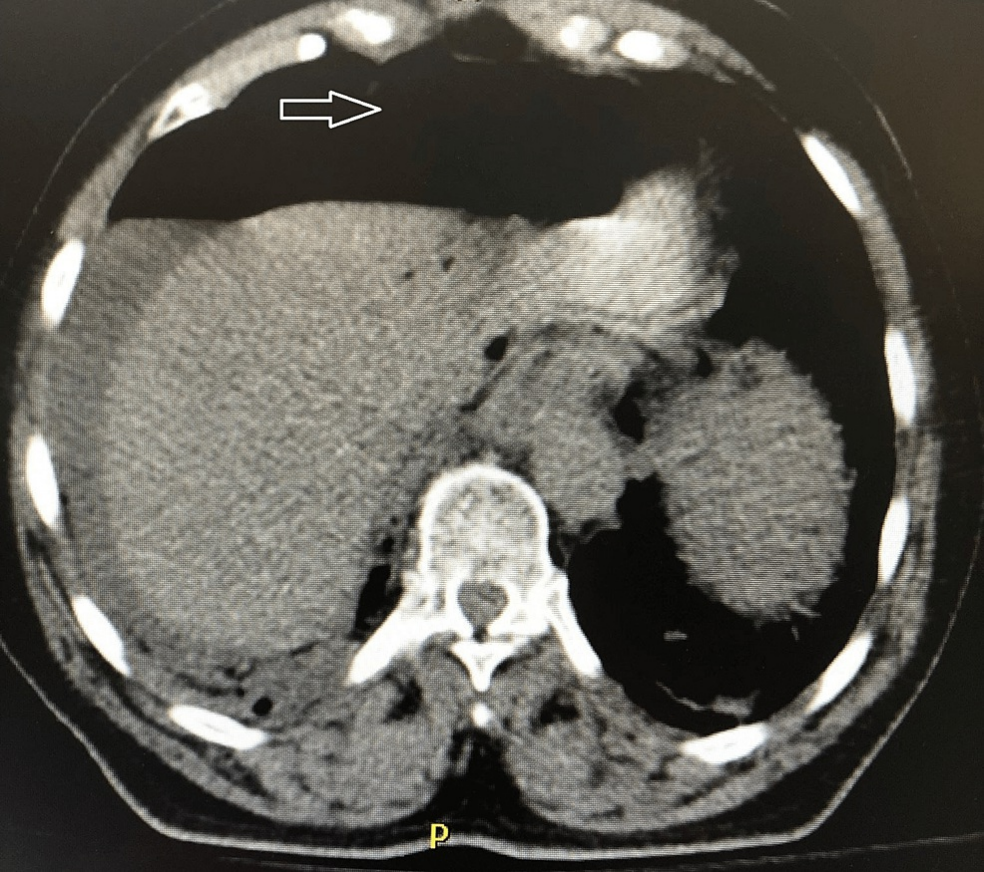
CT showing free air (arrow)
